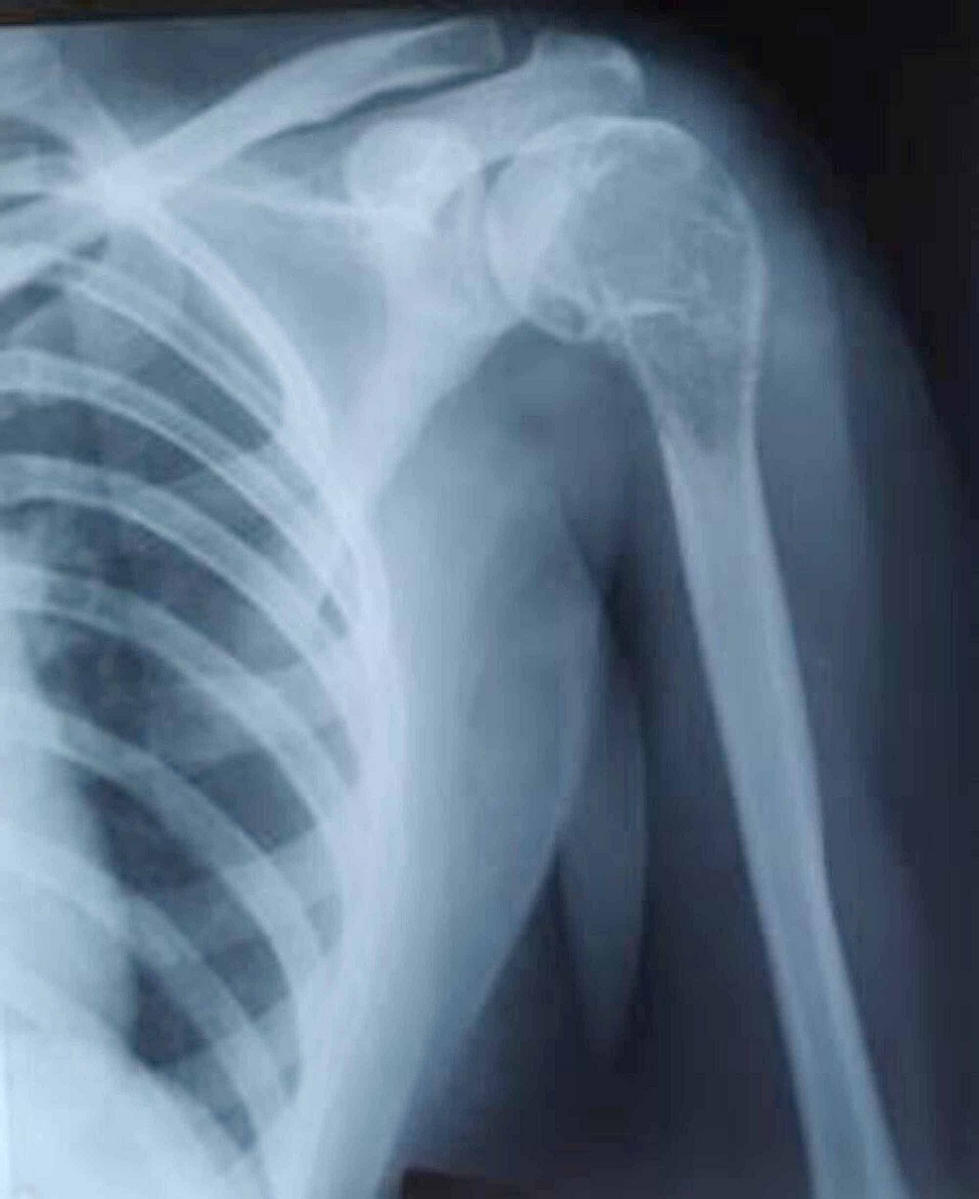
Preoperative X-ray of a giant cell tumour of the left proximal humerus around 20 years ago (2000)
